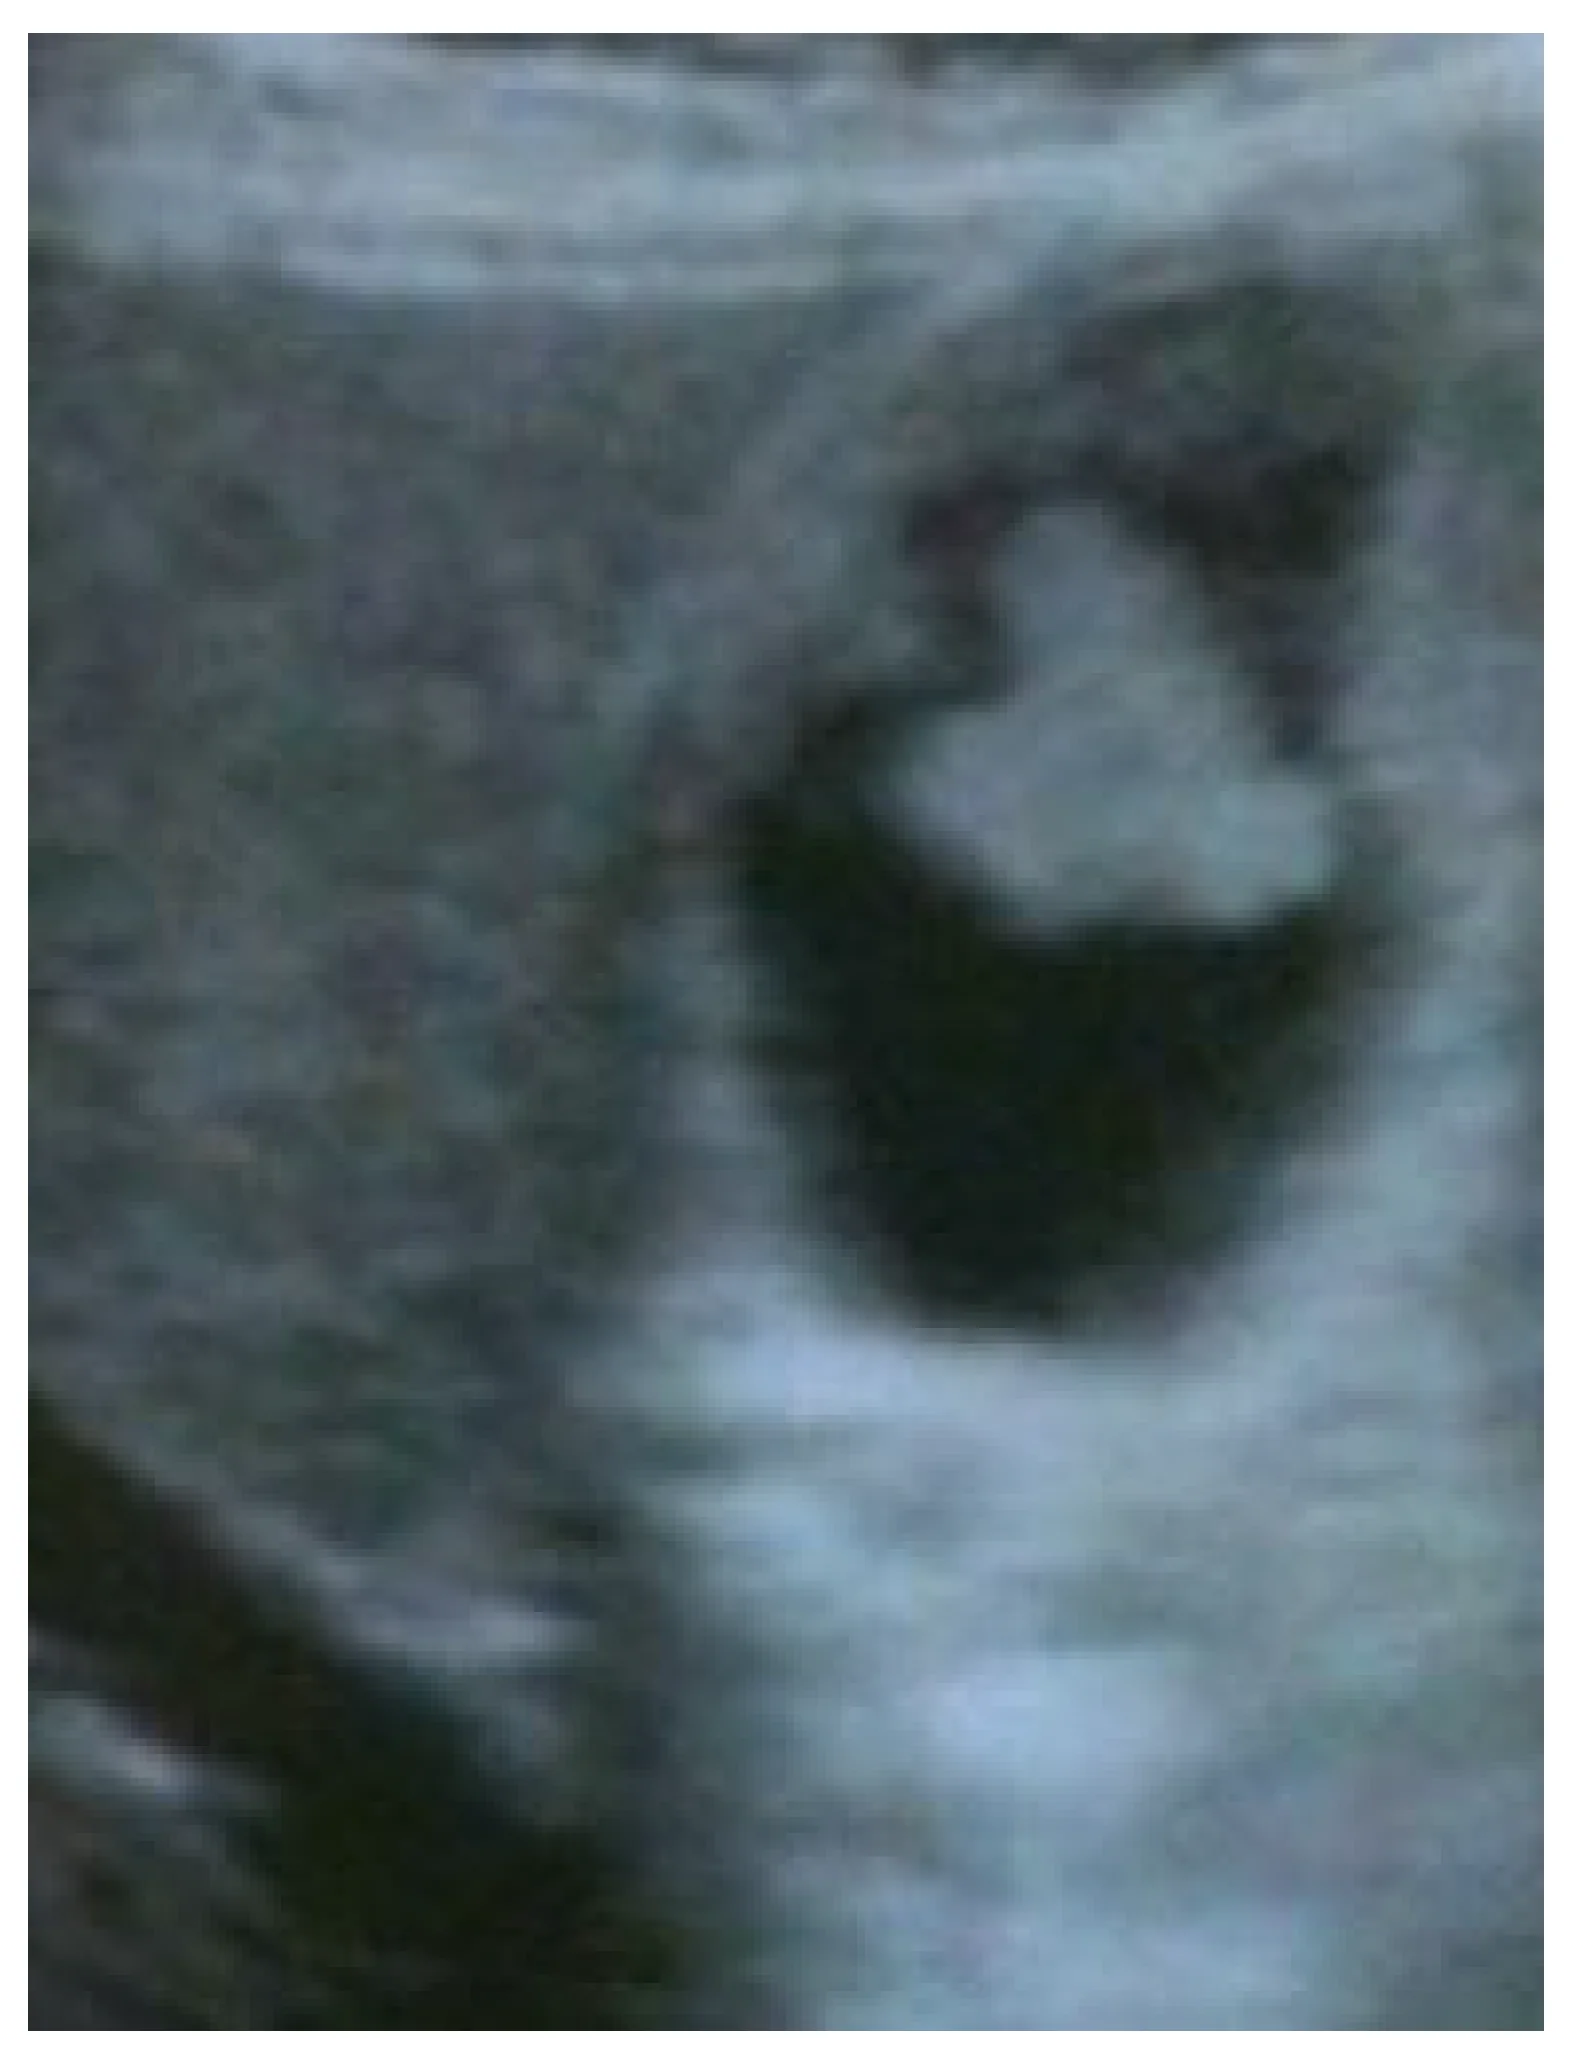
Gallbladder neoplasia is an uncommon cancer that can form from a benign polyp with high-risk features progressing into a malignant mass (e.g., large size > 10 mm with adjacent wall thickening). Gallbladder tumors are often detected incidentally during cholecystectomy or on gallbladder imaging [24,25,26,27]. Surgical pathology is needed for a definitive diagnosis, but ultrasound can be used to visualize and characterize the mass. Figure 7 shows a longitudinal view of a malignant mass present in the gallbladder. Ultrasound is the first-line imaging test for gallbladder malignancies and is used for (1) risk stratification of wall thickening and (2) polypoidal types of gallbladder malignancies. Gallbladder polyps are classified into three categories based on morphologic features and size. Extremely low-risk polyps are pedunculated with a “ball-on-the-wall” character or a thin stalk. Low risk polyps are pedunculated with a thick or wide stalk or a sessile character. Intermediate-risk polyps have adjacent focal wall thickening. A larger sized gallbladder polyp is the strongest predictor for malignancy (e.g., over 10 mm) [24,25,26,27]. In a systematic review, the positive predictive value of transabdominal ultrasound for detecting neoplastic polyps was 4.5% and 1.4% [23]. Yet POCUS users should be cautious because other reviews by Li et al. and Metman et al. found a high false positive rate of 72.5% and 95.4% for transabdominal ultrasound detecting neoplastic polyps [23]. Thus surgical decisions should be based on individual cases and may need additional CT or MRI [21]. Detailed evaluation of gallbladder wall features also helps risk stratify gallbladder malignancies [25,26,27]. A recent systematic review found that presence of echogenic foci, lack of wall disruption, and hypoechoic nodules are associated with benign thickening, while focal thickening and indistinct interface were associated with malignant wall thickening [24,25,26,27]. If the symptoms are debilitating (e.g., severe pain, intractable vomiting, cannot tolerate oral intake, etc.) then the patient may need surgical consultation and hospital admission. Otherwise, they should be referred to oncology for outpatient testing and management.
